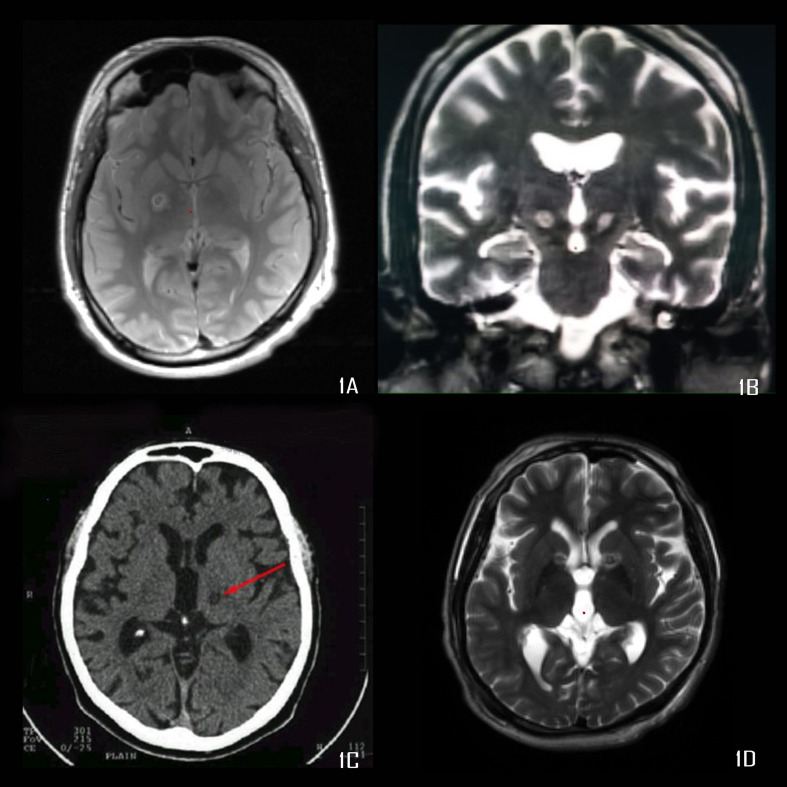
(A) Proton density image showing right pallidotomy, (B) T2 weighted MRI showing bilateral subthalamotomy, (C) CT scan showing Vim thalamotomy, (D) T2 weighted MRI showing bilateral anterior capsulotomy.
